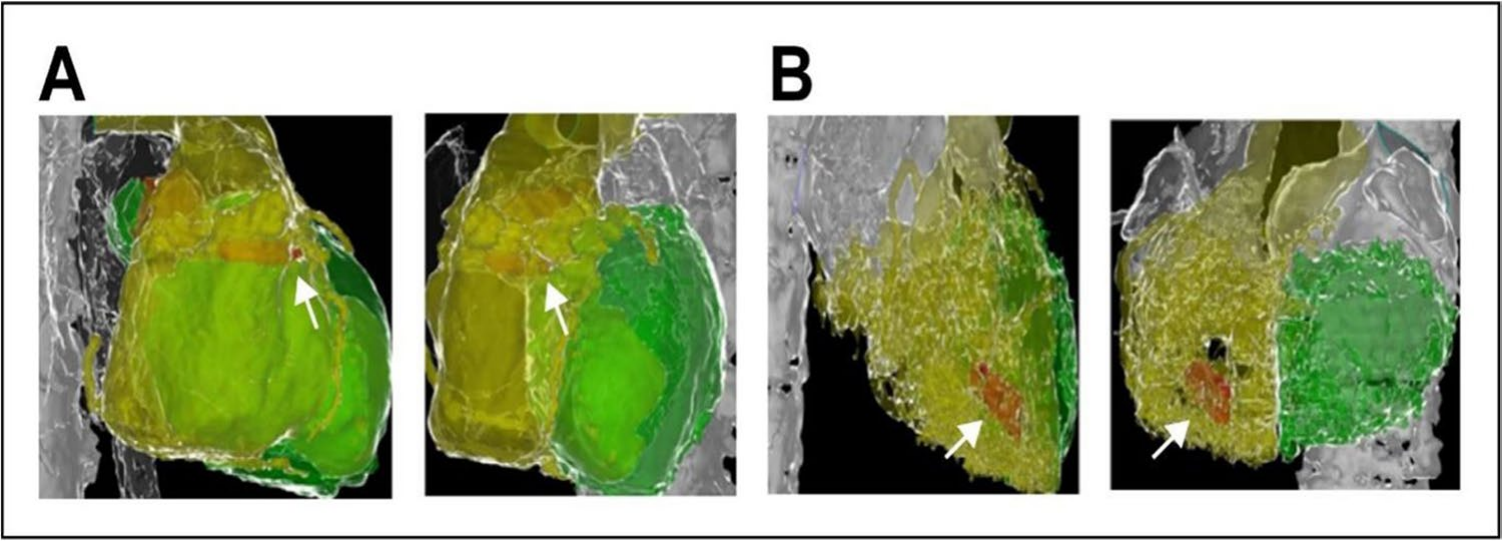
Examples of 3D CT reconstruction of the ventricles and interventricular septum, confirming that the Micra™ (white arrow) in the superior (A, left, RAO30; right, LAO45) and the inferior septum (B, left RAO30, right LAO 45). Yellow: right ventricle; green: left ventricle
